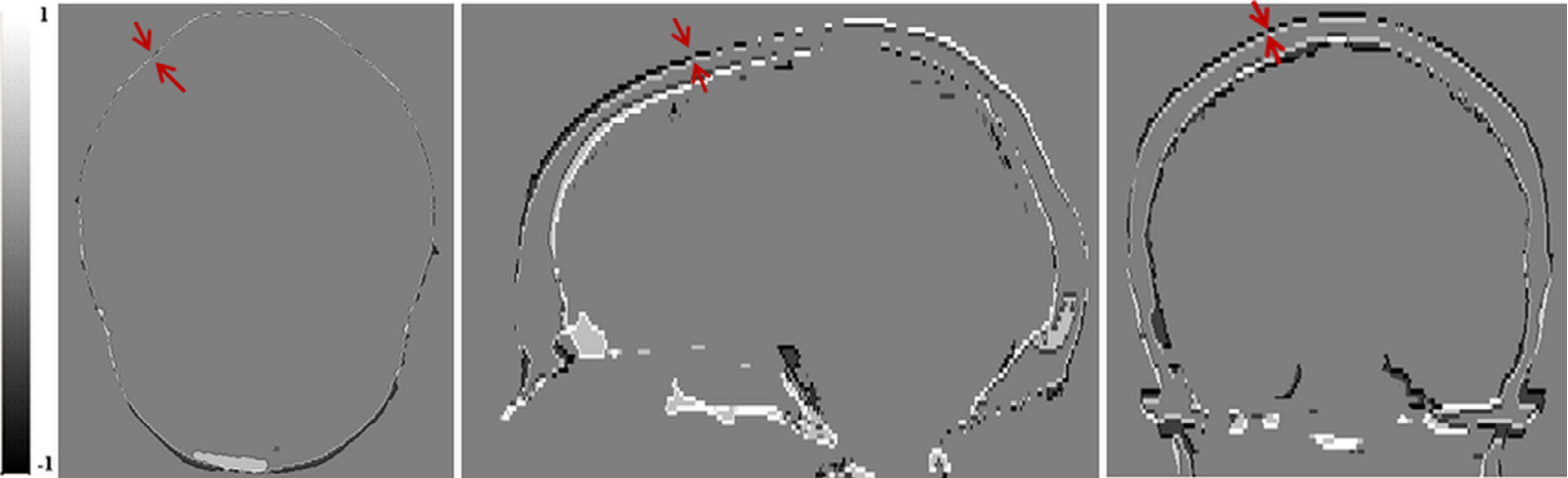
Differences of skull segmentation of synthetic‐computed tomography (sCT) and CT in Axial, Sagittal, and Coronal views. Red arrow indicated the distance between largest external contours of sCT and CT. Black is external skull edge (−1) from sCT and white (1) is external skull edge from CT. Gray is when the edges are overlapped (0).
